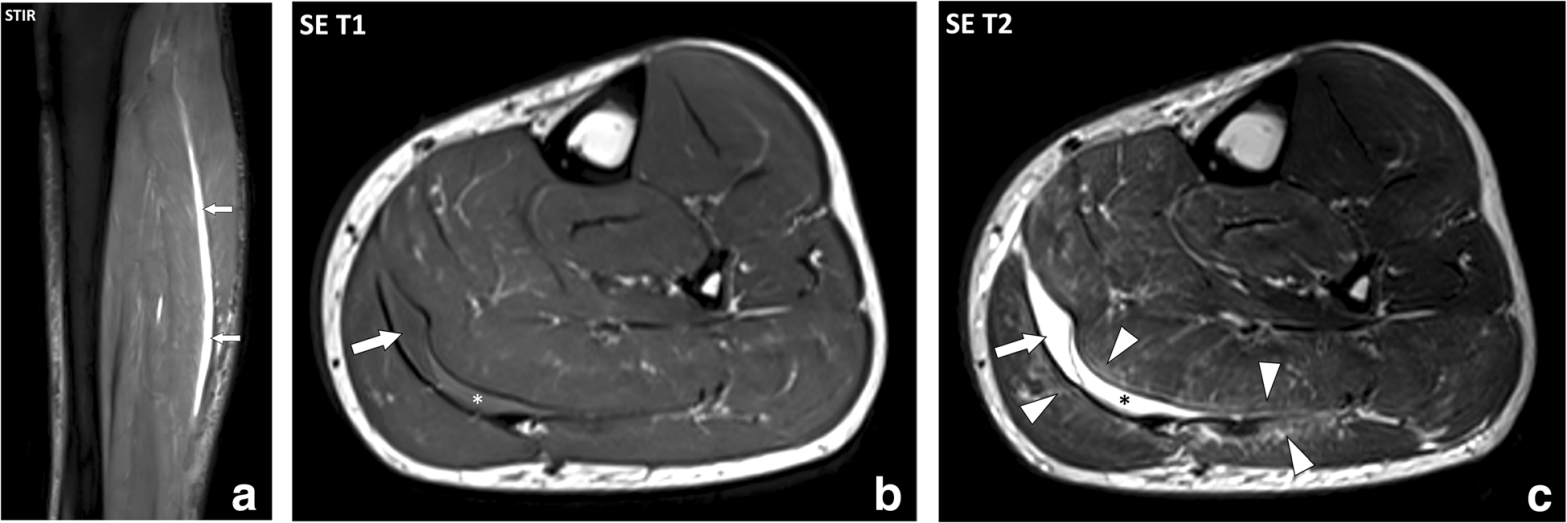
a Sagittal STIR, (b) axial SE T1w, and (c) axial SE T2w images of the left leg of a 41-year-old male with myofascial injury of the calf after a skiing accident. MRI demonstrates a fluid collection at the interface between the medial head of the gastrocnemius muscle and the soleus muscle (arrows) with a component of intermediate signal intensity on the T1w image corresponding to blood (asterisks). Note the infiltration of the connective tissue of the muscles adjacent to the collection (arrowheads)
